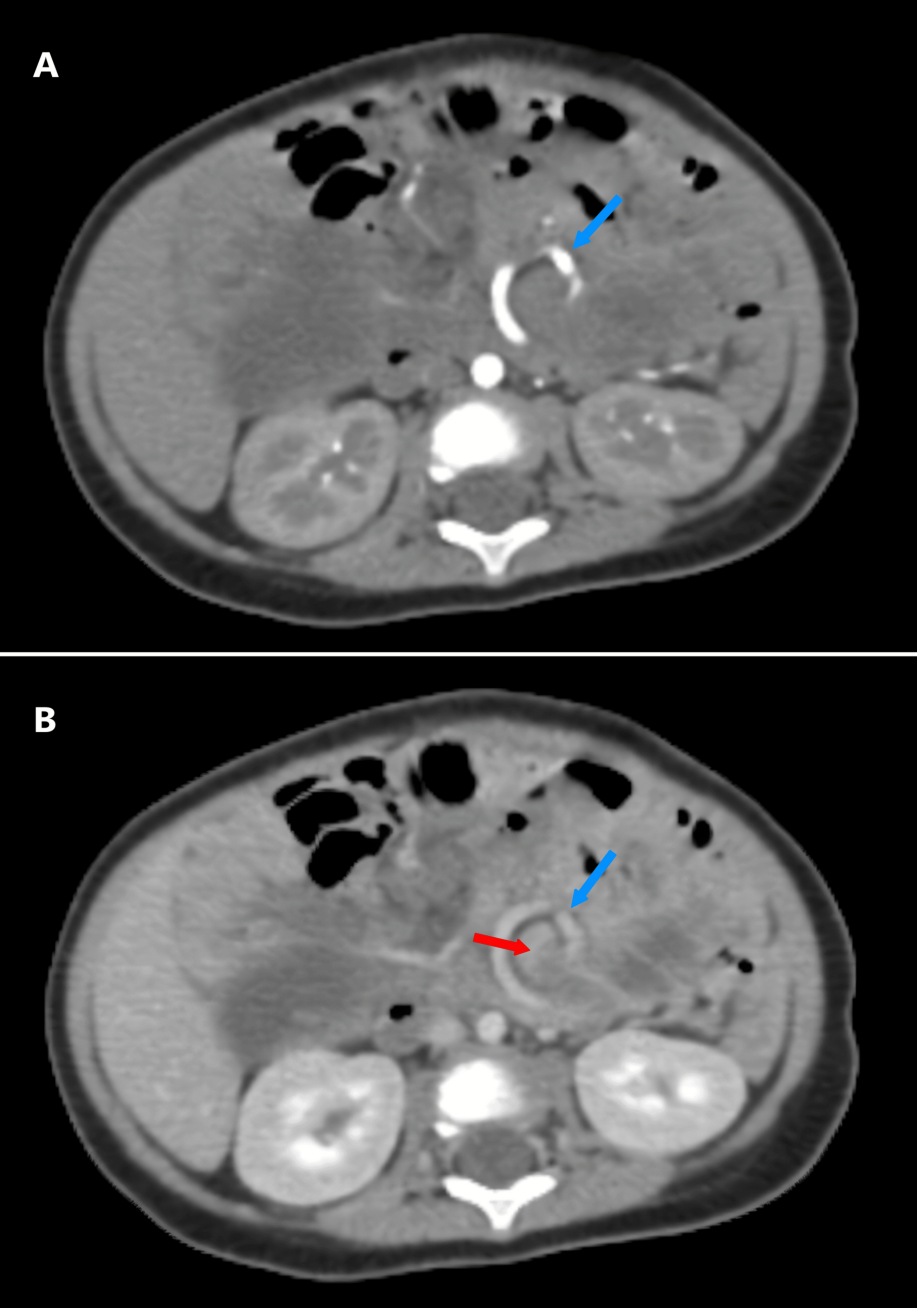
Axial CT scan findings A: arterial phase; B: venous phase showing the “whirlpool sign” pathognomonic of mesenteric volvulus (red arrow: twisted intestinal loop, blue arrow: mesenteric vessels).
